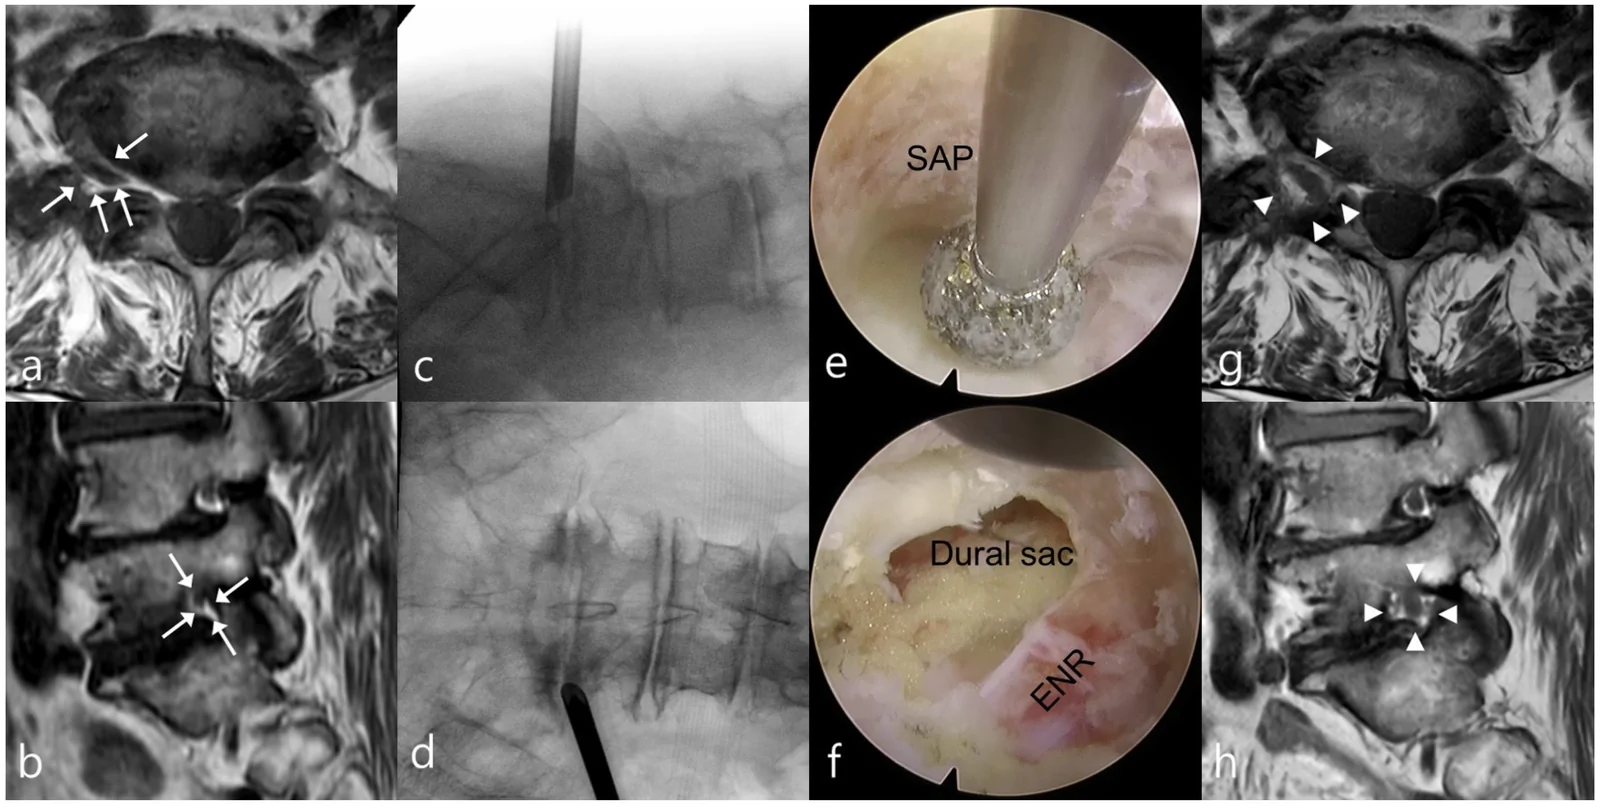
Representative case of TELF for lumbar foraminal stenosis. Preoperative axial and sagittal magnetic resonance imaging (MRI) images demonstrate severe foraminal stenosis caused by hypertrophy of the superior articular process (SAP) and ligamentum flavum (arrows) (a,b). Intraoperative fluoroscopic images show the position of the working sheath (c,d). Endoscopic views demonstrate SAP resection (e) and exposure of the exiting nerve root (ENR) and axillary epidural space after decompression (f). Postoperative axial and sagittal MRI images demonstrate adequate foraminal decompression (arrowheads) (g,h).
